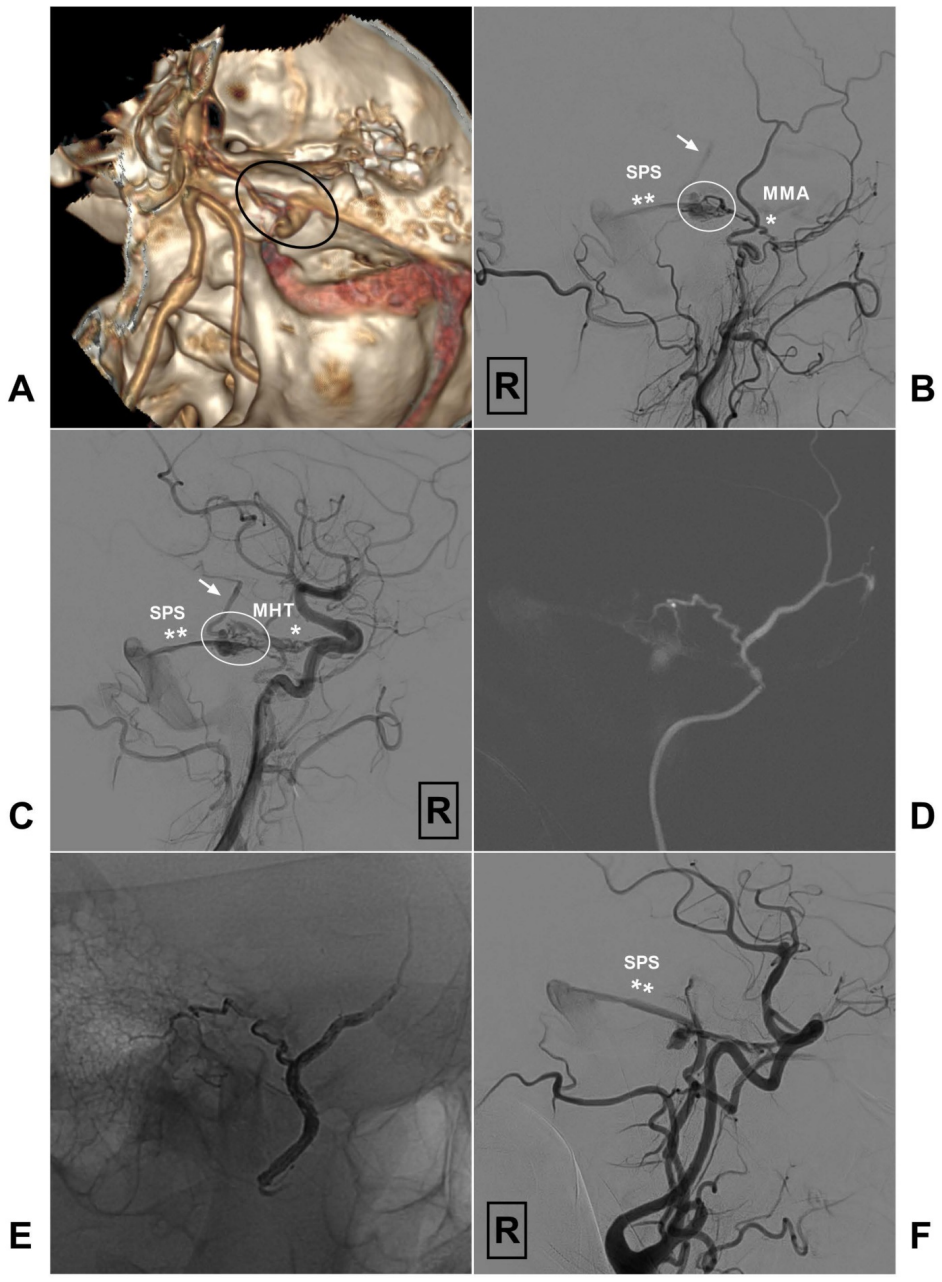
A, CTA reveals a petroclival DAVF (encircled area). B, Angiogram of the right ECA in lateral view shows the DAVF (encircled area) is supplied by the MMA (single asterisk) and drains to the SPS (double asterisks). Besides, retrograde venous drainage (arrow) to the brainstem is also noted. C, Angiogram of the right ICA in right anterior oblique view shows the DAVF (encircled area) also receives blood supply from the MHT (single asterisk) and drains to the SPS (double asterisks). Retrograde venous drainage (arrow) to the brainstem is also noted. D, Super-selective angiogram of the right MMA shows the branch supplying the DAVF is tortuous and enlarged. E, Onyx could not penetrate the fistula point during transarterial embolization of the DAVF via the MMA. F, Angiogram of the ICA shows the DAVF still has residual venous drainage via SPS (double asterisks). Abbreviations: CTA, computed tomography angiography; DAVF, dural arteriovenous fistula; ECA, external carotid artery; ICA, internal carotid artery; MHT, meningohypophyseal trunk; MMA, the middle meningeal artery; R, right; SPS: superior petrosal sinus.
